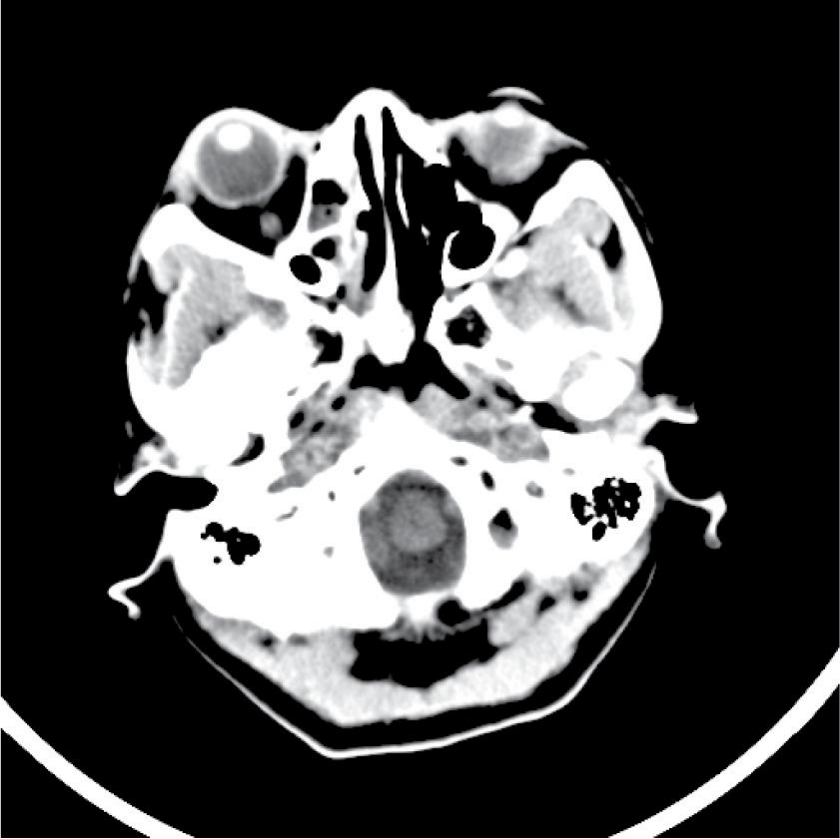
Case 1, computed tomography (CT) with partial occupation of right ethmoidal cells and right sphenoid sinus.
